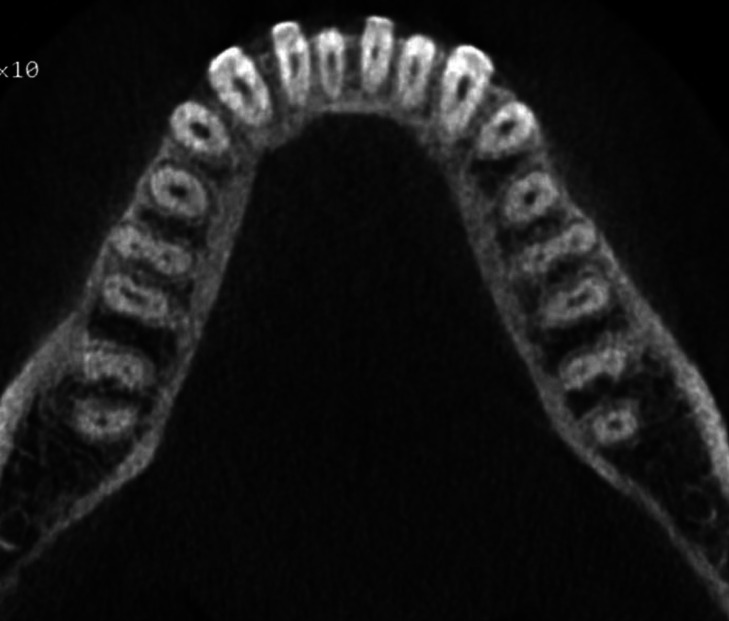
Cross-sectional cone-beam computed tomography image of a mandibular second molar with presence of two roots and three canals occurring bilaterally.
